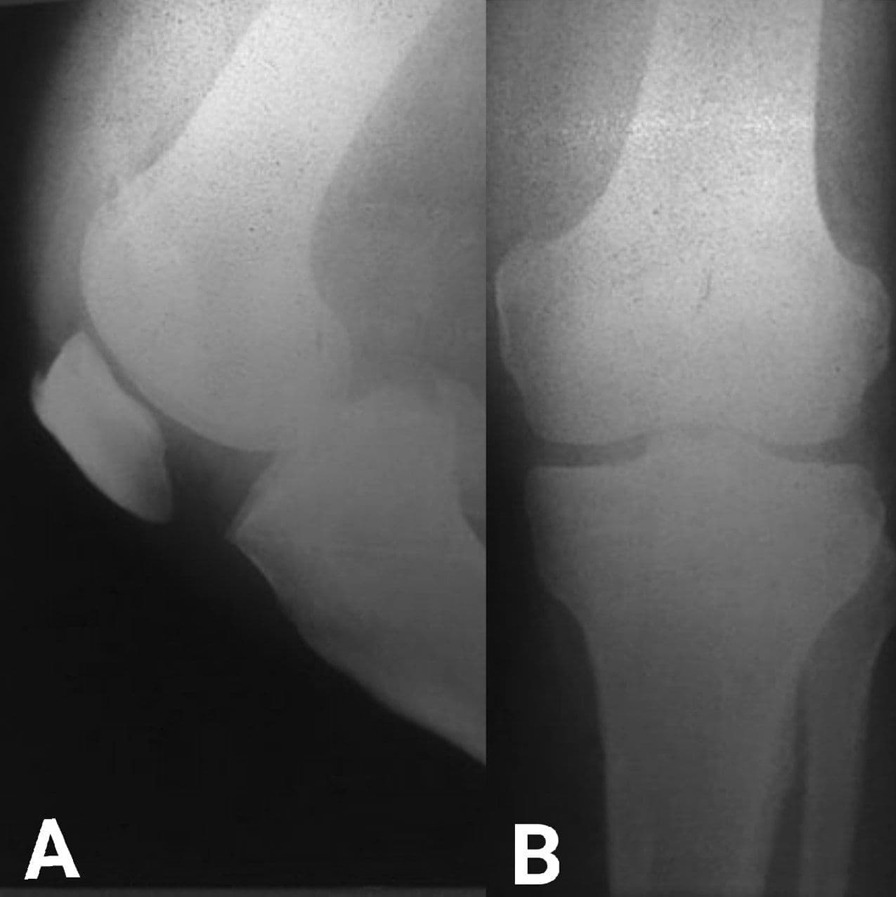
A, B: Pre-operation plain X-ray shows mild degenerative changes
